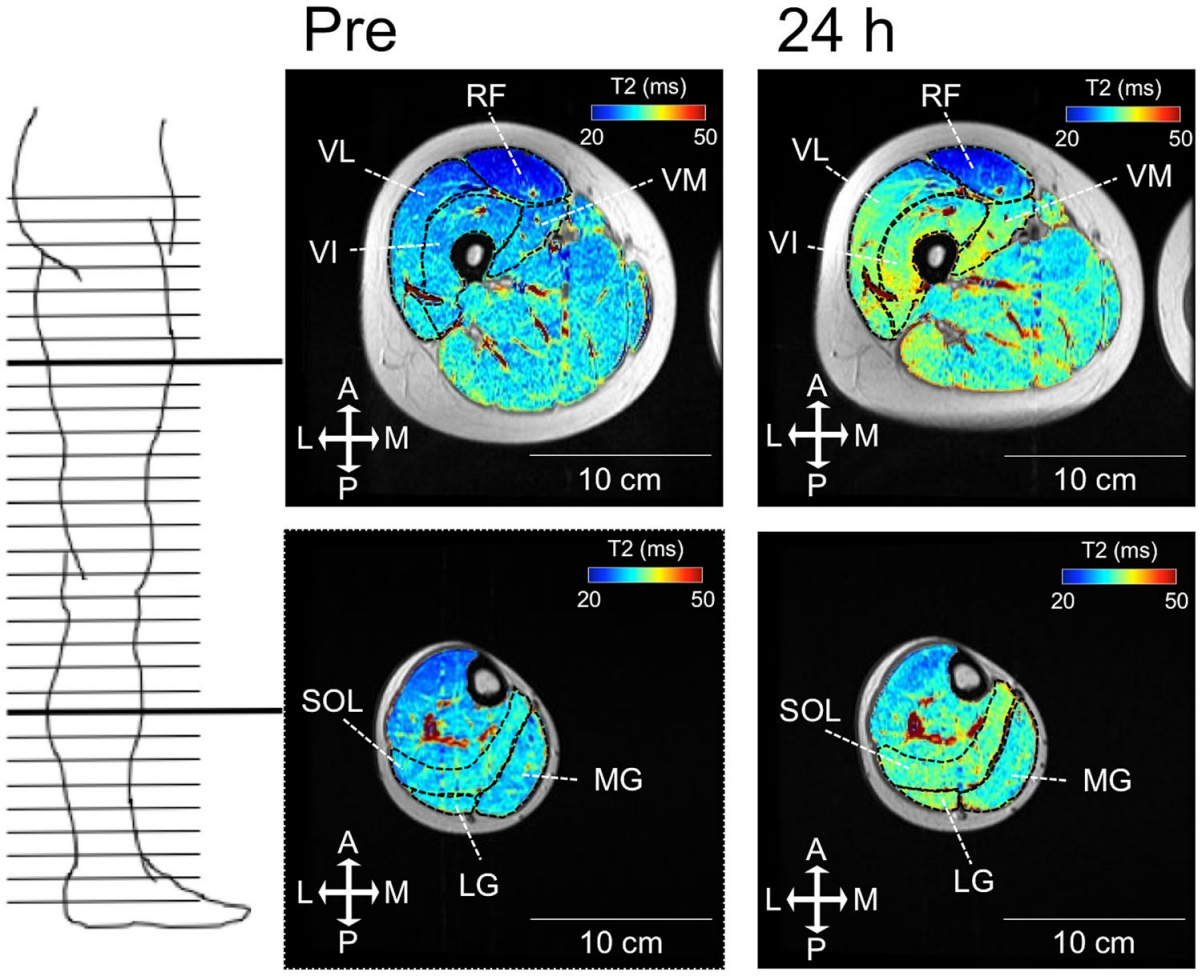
Examples of T_2_ maps superimposed on T_2_-MRIs at middle sites for the VI (KEs, upper figures) and SOL (PFs, lower figures) scanned before (pre, left) and 24 h after (right) the downhill running task for one participant are shown. T_2_: transverse-relaxation time, MRI: magnetic resonance imaging, RF: rectus femoris, VI: vastus intermedius, VL: vastus lateralis, VM: vastus medialis, LG lateral gastrocnemius, MG medial gastrocnemius, SOL: soleus, A, M, P, L: anterior, medial, posterior, and lateral side, respectively.
